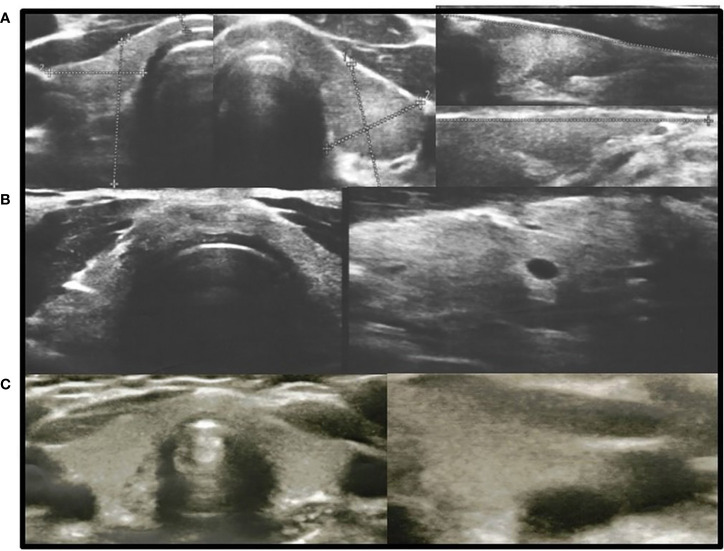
Axial and longitudinal ultrasonography scans of the thyroid gland in three patients with hypothyroidism presenting with decreased echogenicity and irregular thyroid structure. Blood flow is normal. (A), Patient H1; (B), Patient D1; (C), Patient M1. Samsung HS40.
